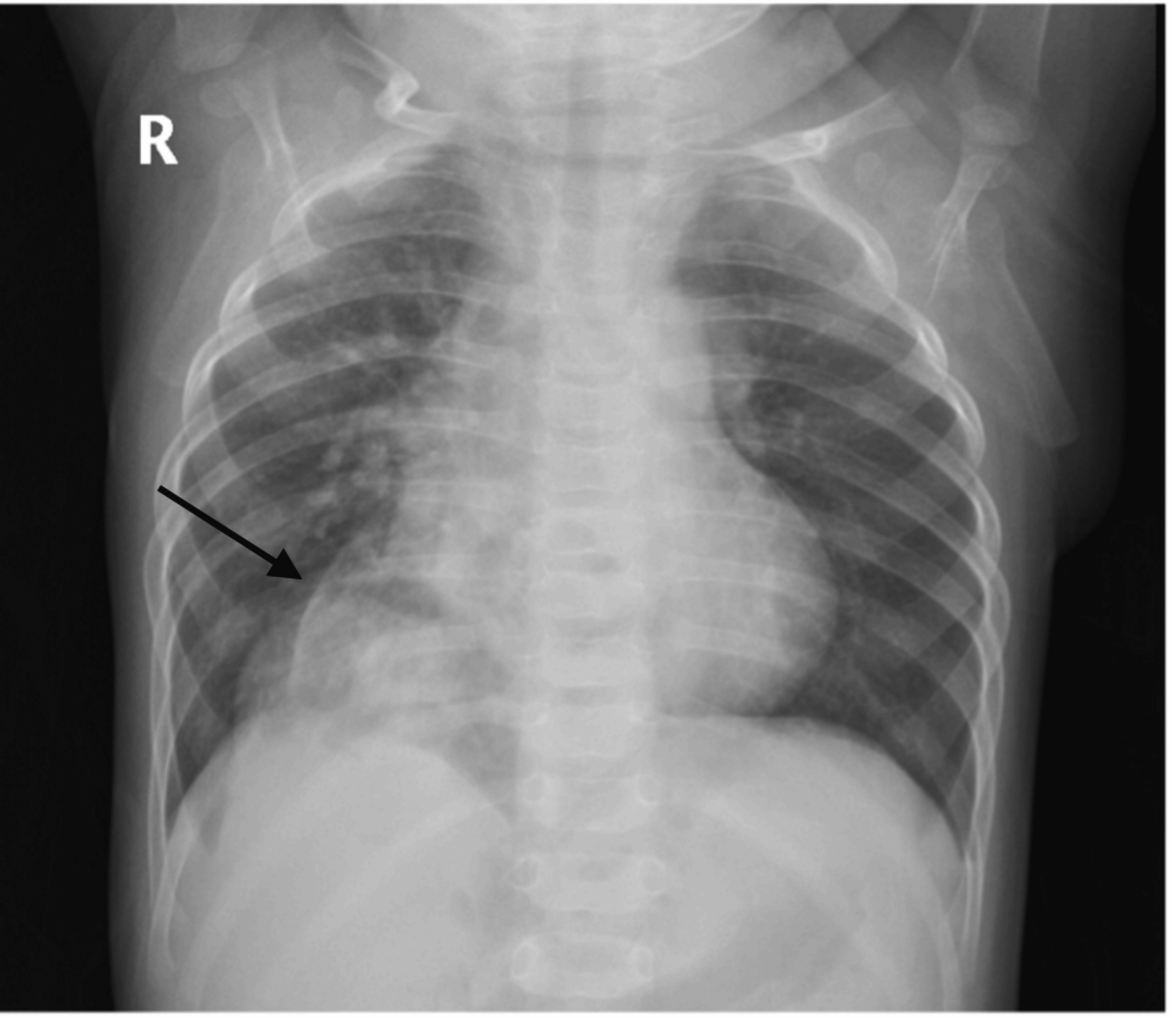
Chest radiograph. The image reveals an abnormal radiolucent area in the right cardio-phrenic angle, containing air-filled bowel loops, consistent with herniated abdominal viscera.
